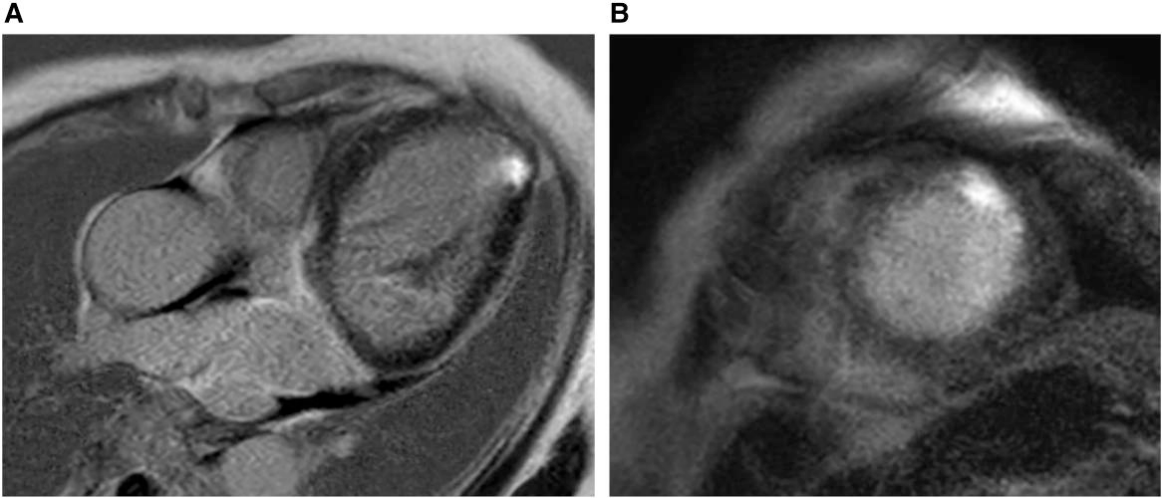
CMRI reveals LGE at the endocardial side of the left ventricle's anterior wall about (*A*) long axis view and (*B*) short axis view. CMRI, gadolinium-enhanced cardiovascular magnetic resonance imaging; LGE, late gadolinium enhancement.
